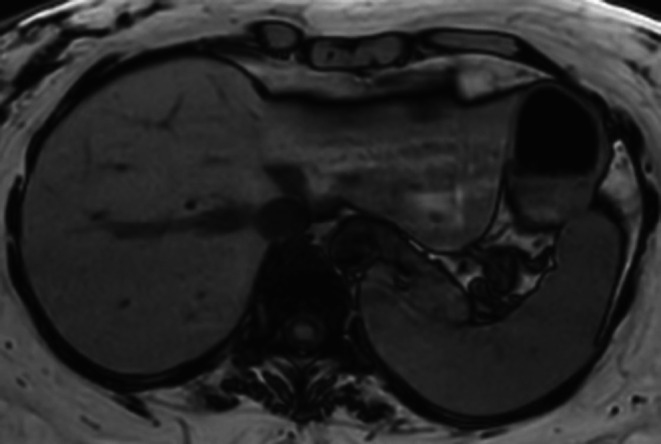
Magnetic resonance imaging (MRI; T1‐weighted duo‐echo out‐of‐phase) presented in an axial plane reconstruction from day 180 after hospital admission. The protocol also included Dixon fat quantifying sequences estimating hepatic fat content to be 3%–4%.
